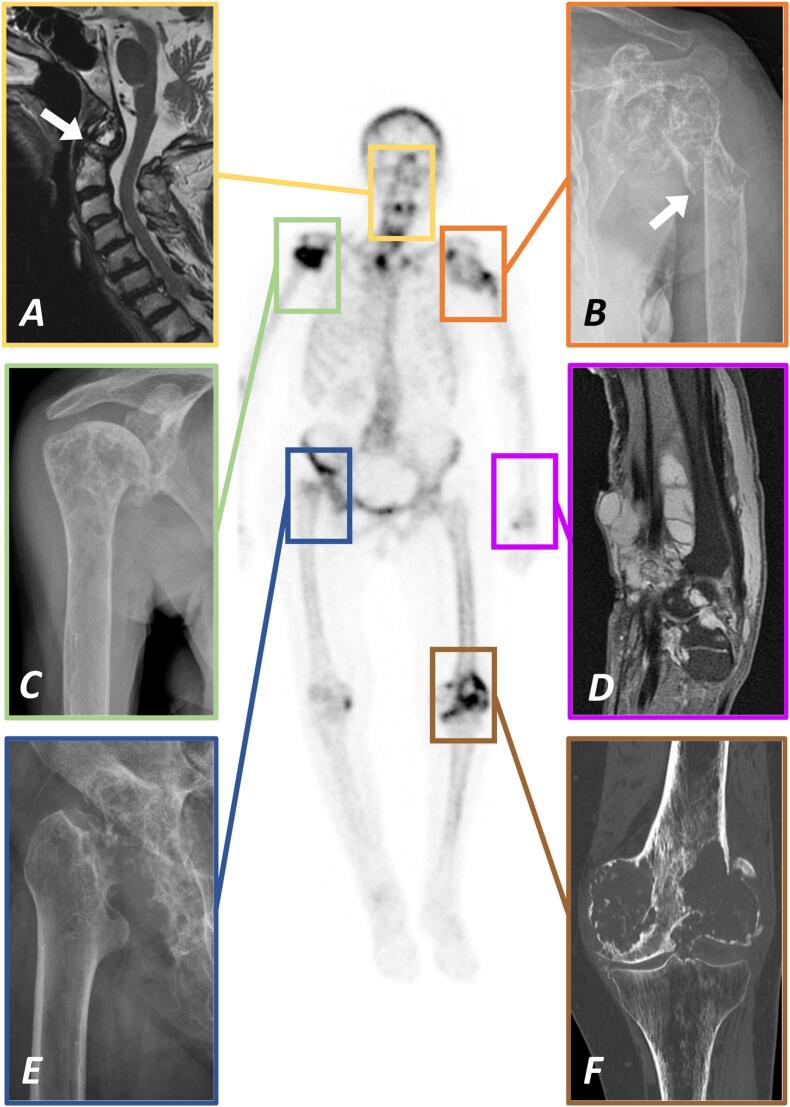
Bone scintigram showing the predominant skeletal manifestations of multifocal osteolyses in the 83-year-old female patient. Note the osteolytic destruction and undisplaced fracture of the dens (A; MRI T1 sagittal; white arrow pointing at the fracture site) which required fusion of C1 and C2 and the consolidated fracture of the left proximal humerus diaphysis following radiation therapy (B; X-ray anteroposterior; white arrow pointing at the fracture site) which was treated conservatively. The osteolysis of the right proximal humerus (C; X-ray anteroposterior) as well as the polycystic tumor surrounding the extensor tendons at the left wrist (D; MRI PD FS sagittal) did not receive any specific treatment. Radiation therapy was applied to the right hip joint (E; X-ray anteroposterior) as well as to the left distal femur and knee joint following distal femoral replacement due to a pathological fracture (F; CT coronal).
